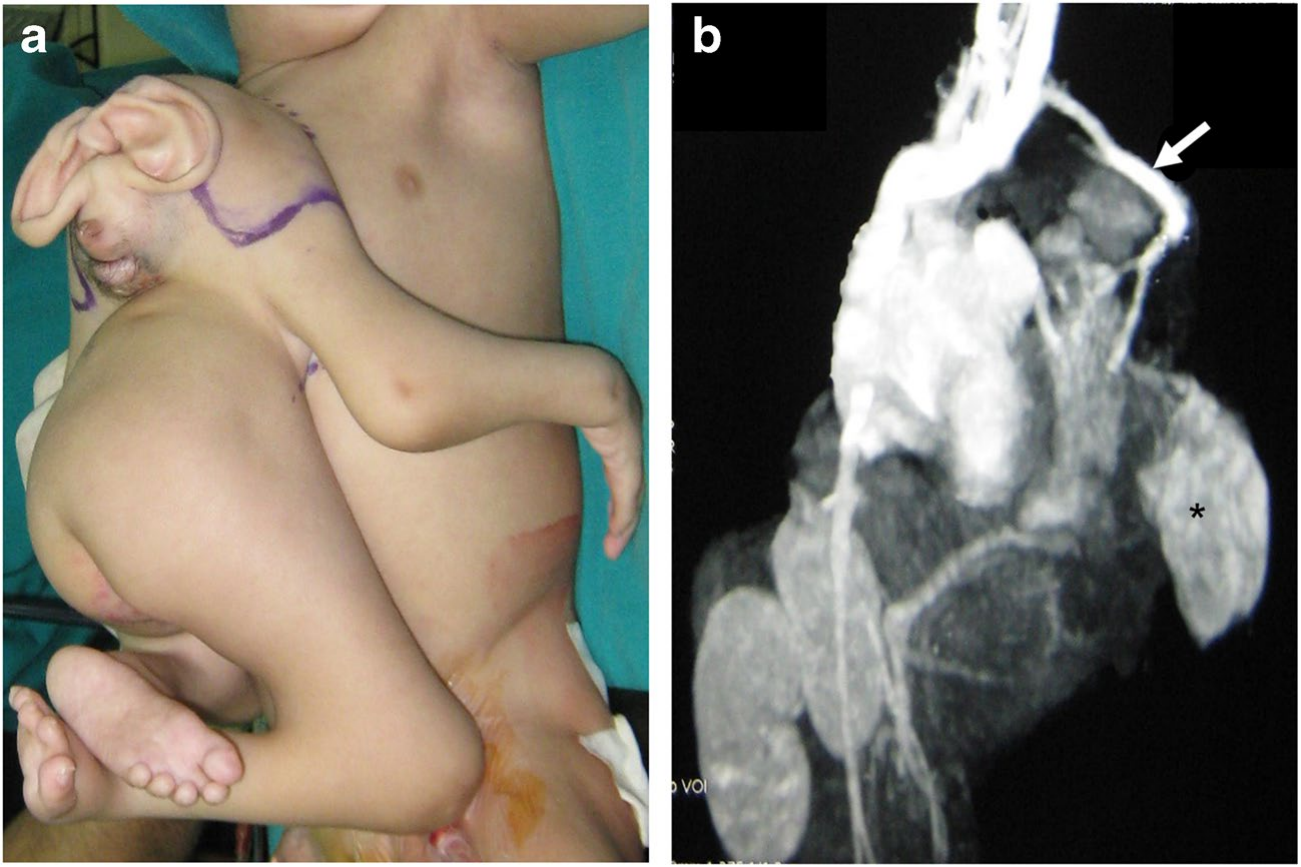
15-month-old heteropagus conjoined twin girls. a A photographic images shows the parasitic twin attached to the anterior chest wall of the autositic twin. The parasitic twin has a deformed cranium and incomplete trunk with four limbs. b Maximum intensity projection computed tomography angiography shows an anomalous vessel (*arrow*) supplying the parasitic twin which originates from the subclavian artery of the autosite. Note the kidney of the parasitic twin (*asterisk*)
